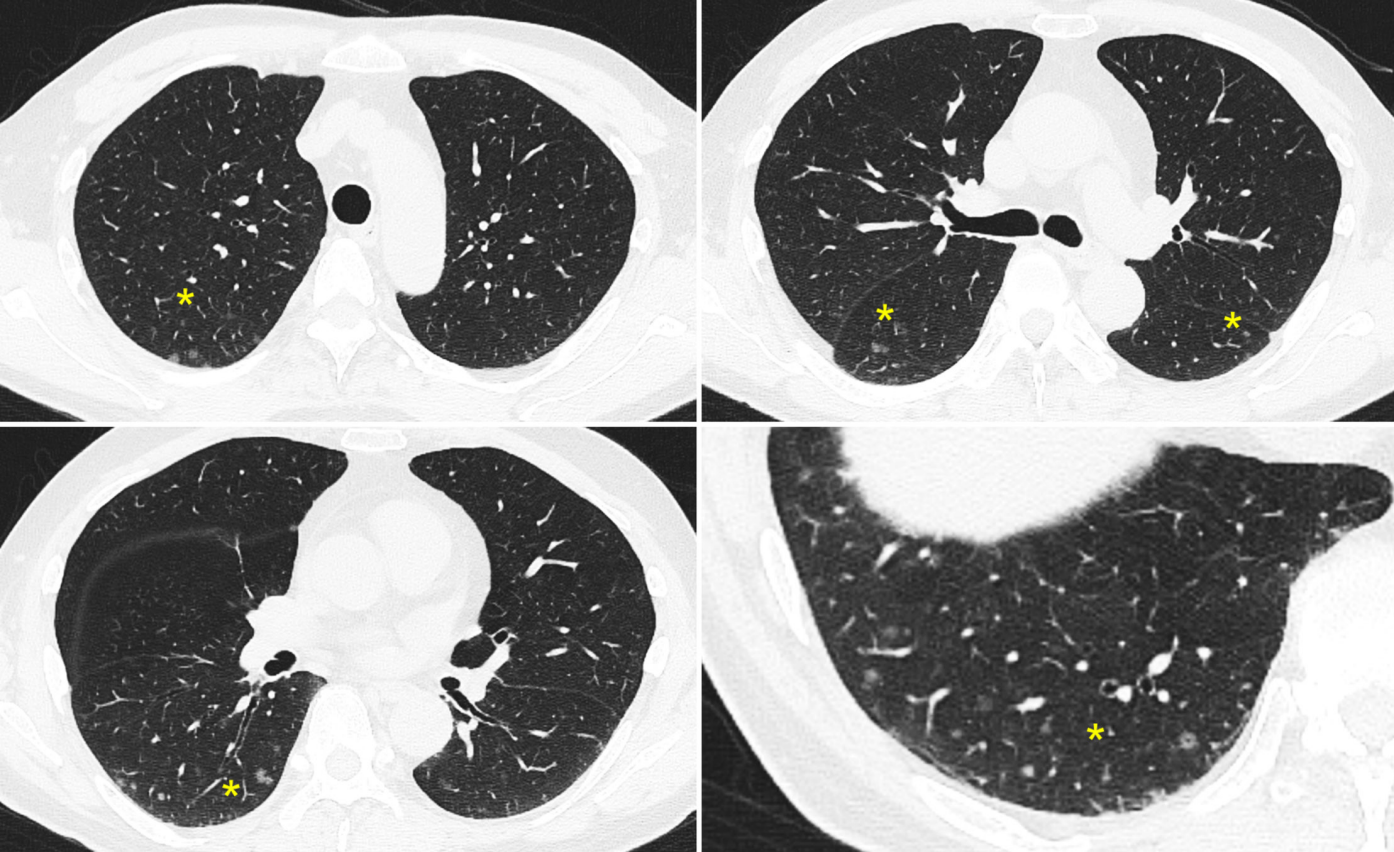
High‐resolution chest computed tomography showed multiple diffuse small ground‐glass nodules at the peripheral zone of each lung (yellow asterisks), some of which demonstrate central cavitation.
